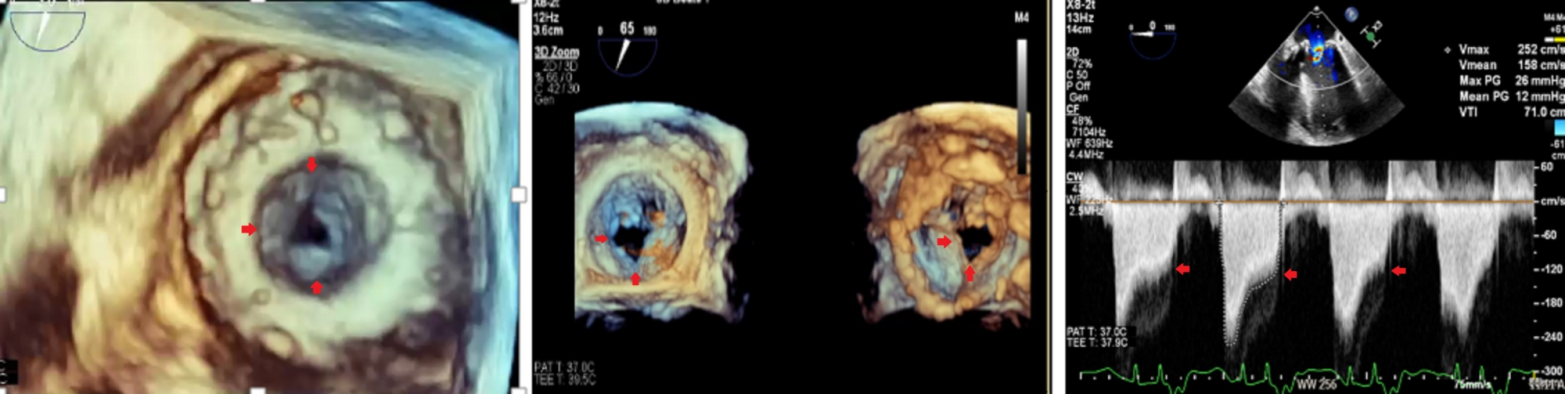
Post-transcatheter mitral valve-in-valve replacement transesophageal echocardiogram normal gradients across the aortic valve and normal valvular excursion in systole
